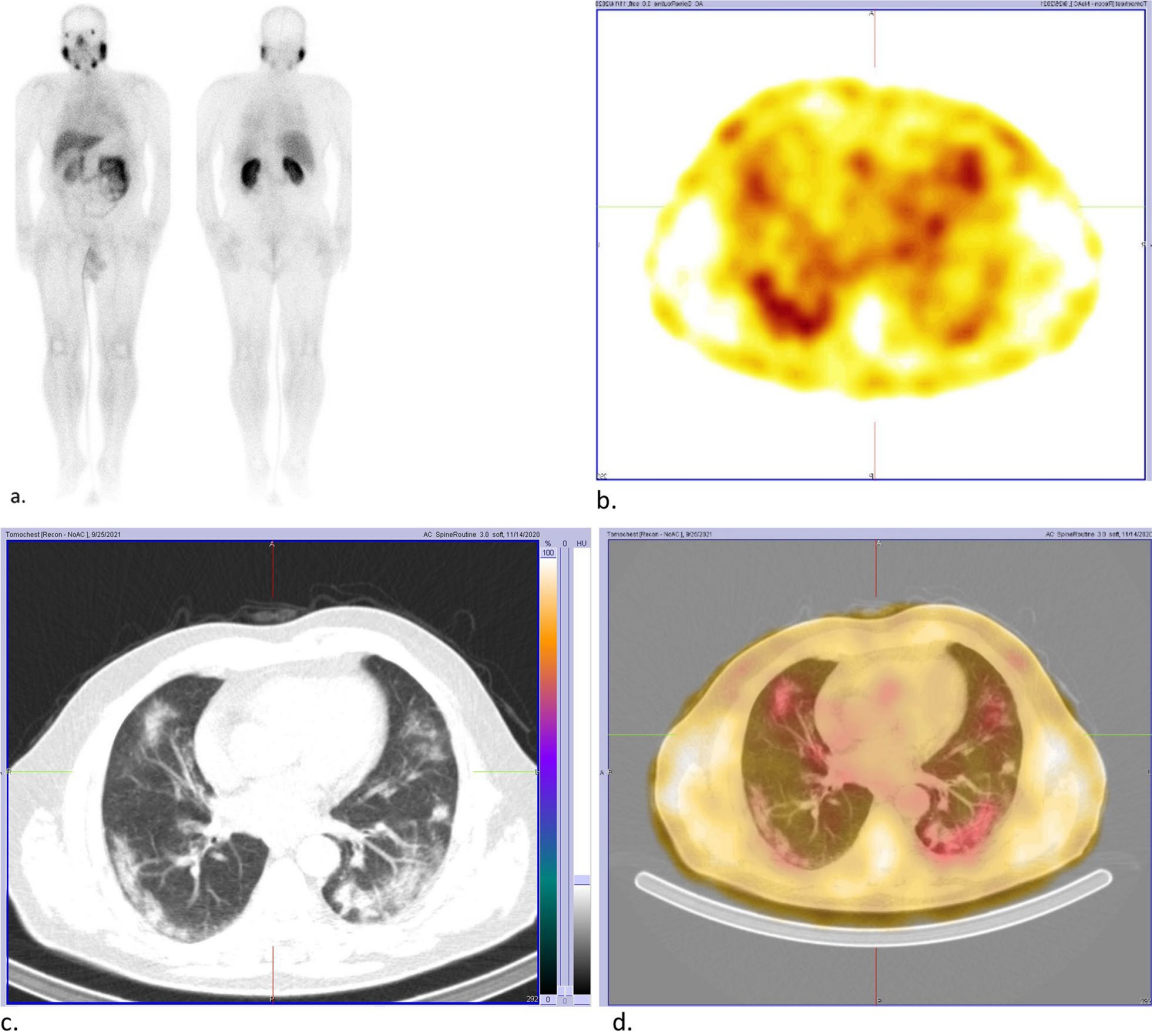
a Whole-body 99mTc-PSMA study with faint uptake in the chest region as well as physiologic distribution of radiotracer. b SPECT reveals mild peripheral 99mTC-PSMA uptake in the lung fields. c CT shows multifocal, bilateral and peripheral ground-glass opacities in lungs. d Fused SPECT/CT shows the PSMA uptake is matched by ground-glass opacities in the lung (left lung COVID-related consolidation also shows the same uptake just it is not apparent in this cut due to respiratory motion misregistration)
